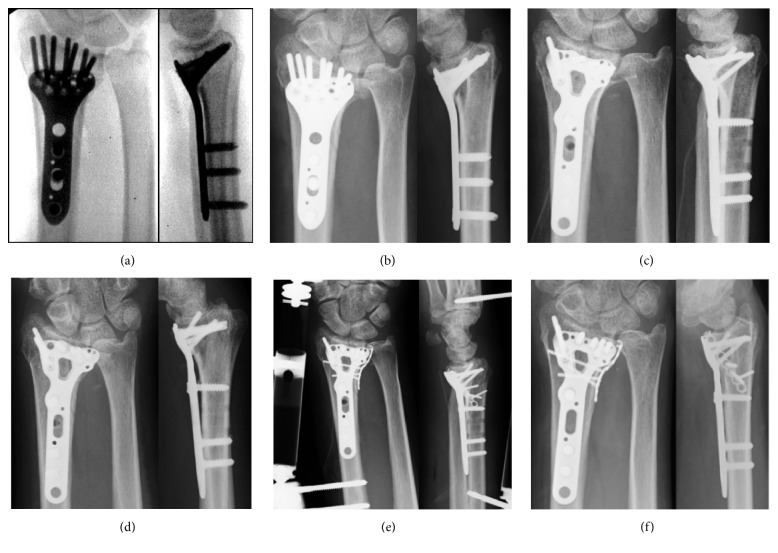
Plain radiographs of Patient 1. (a) Immediately after the first surgery. (b) Two weeks after the first surgery; redisplacement is seen. (c) Immediately after reoperation. (d) Five days after reoperation; redisplacement is seen. (e) Immediately after the third surgery. (f) One year after the third surgery; bone union was achieved without redisplacement.
